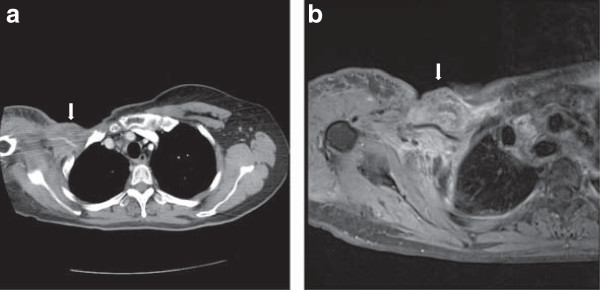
Preoperative coronal CT (a) and T1-weighted MRI (b) images of the tumor lesion surrounding the brachial plexus over the right axillary area with chest wall invasion (arrow).
